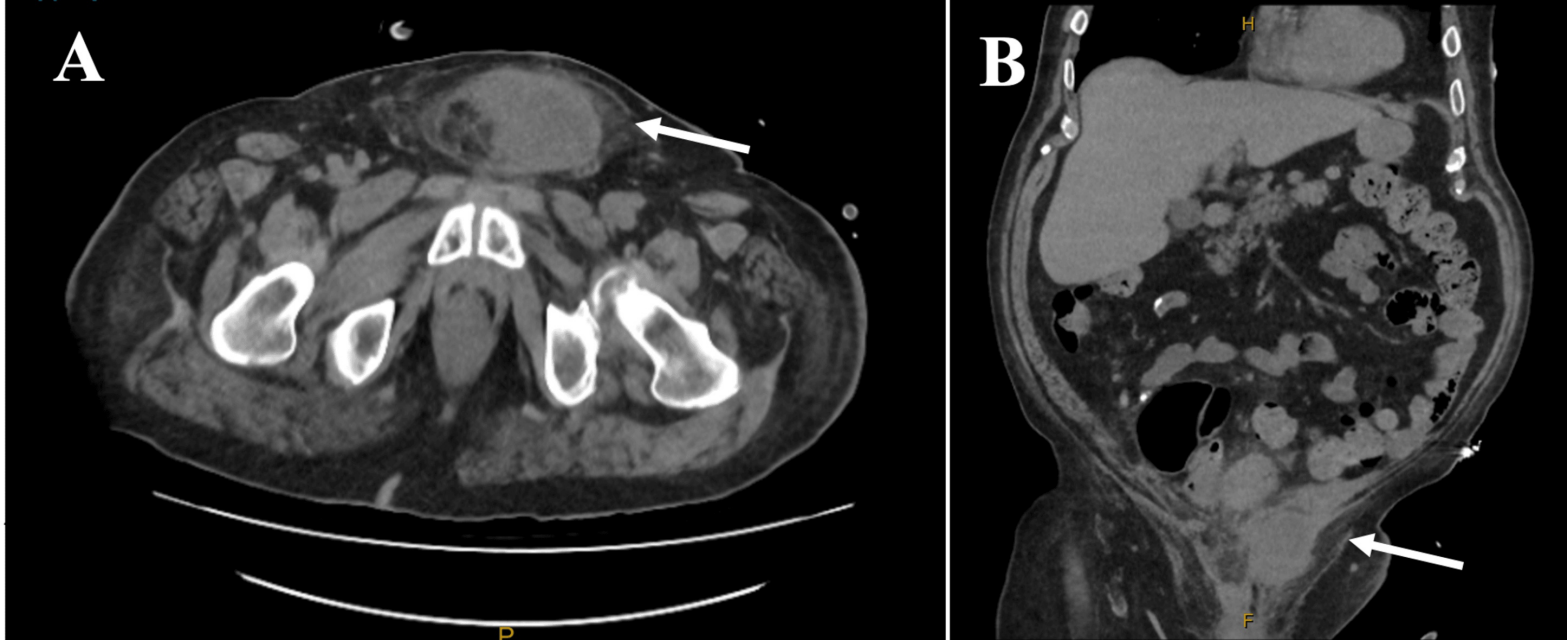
Contrast-enhanced CT of the abdomen and pelvis demonstrating a suprapubic abscess with suspected colocutaneous fistula secondary to sigmoid diverticulitis. (A) Axial CT image shows a thick-walled, peripherally enhancing suprapubic collection located slightly left of midline, abutting the dorsal margin of the penile base (white arrow). (B) Coronal CT image demonstrates a well-defined tract extending superiorly from the suprapubic collection to the inferior aspect of the sigmoid colon (white arrow), which shows associated mural thickening and pericolic fat stranding consistent with diverticulitis. No gas is seen within the collection, and there is no pneumoperitoneum, supporting a contained inflammatory fistula rather than free perforation.
